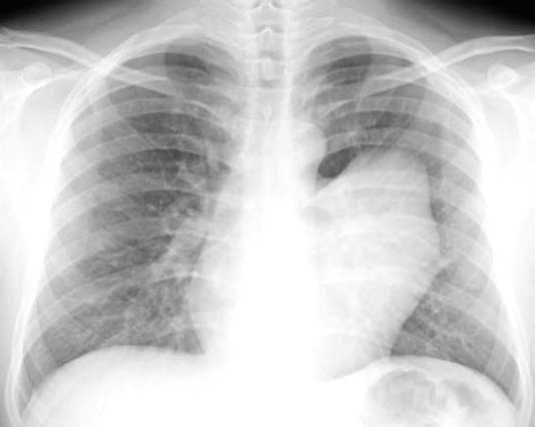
Chest X-rays showing mediastinal mass. Chest X-ray showing a large round opacity of the left hilum.
